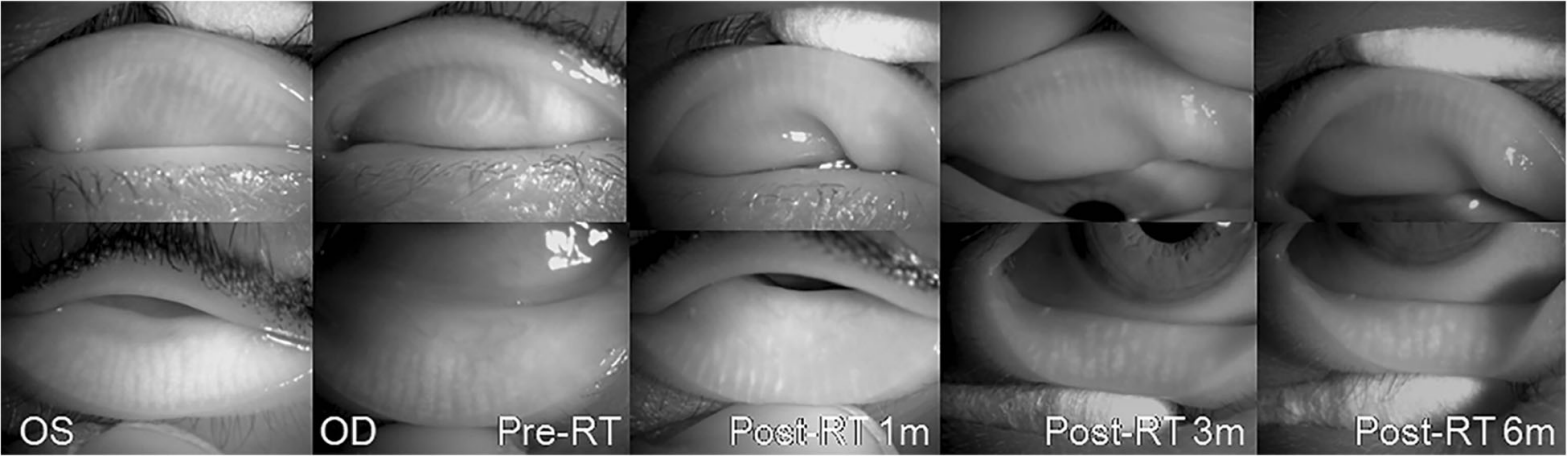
Comparison of the meibomian glands of irradiated eye with the fellow non-irradiated eye. A 54-year-old female patient with lacrimal gland mucosa-associated lymphoid tissue lymphoma in the right eye received a radiation dose of 3060 cGy and 6 MV X-ray. Note the distortion, shortening and dropout of meibomian glands in the irradiated eye
